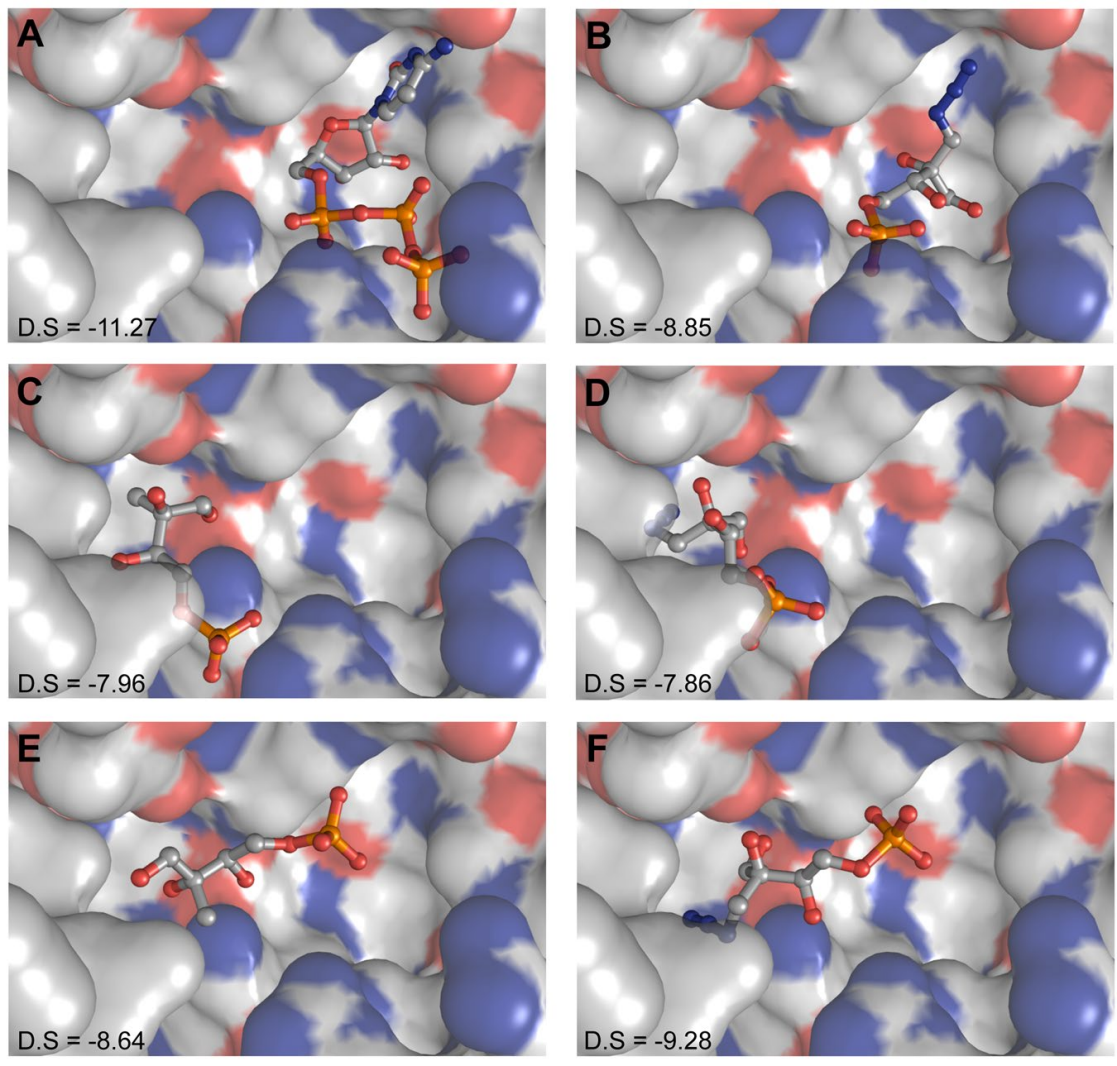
In silico* docking results. Docking experiments were performed with the X-ray structure of *E*. *coli* YgbP: CTP complex (PDB ID: 1I52). The best docking poses and their corresponding docking scores (D.S) are reported. (A,B) CTP and respectively MEPN_3_ docked using a target with an empty binding pocket. (C) to (F) docking poses revealing the two possible binding sites observed for MEP (C,E) or MEPN_3_ (D,F) when the docking experiments were performed with a target already containing bound CTP. The compounds were docked using Glide in extra precision (XP) mode and the Glide docking score was used to rank the docking poses.
